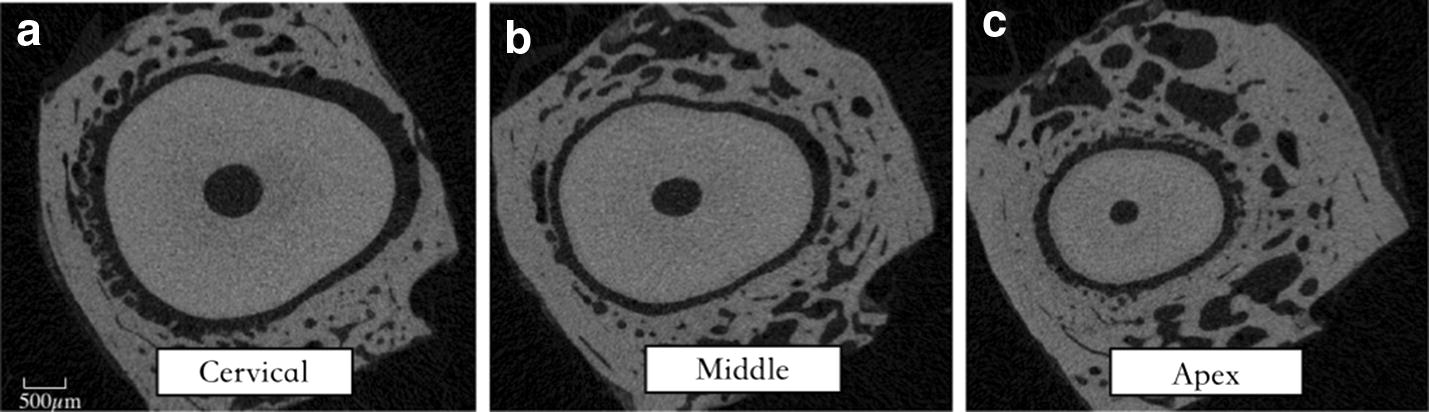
Tooth imaged by micro CT. a–c Section images of cervical, middle, and apical root level of human PDL
